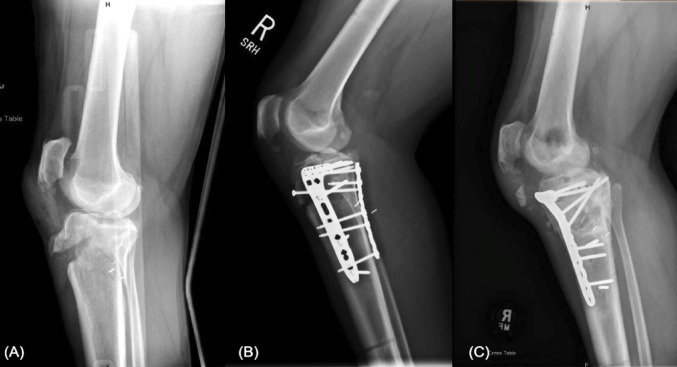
Representative case of fixation failure after operative treatment of a tibial tubercle–involving tibial plateau fracture. A Preoperative lateral radiograph. B Immediate postoperative lateral radiograph demonstrating the index fixation construct. C Follow-up lateral radiograph demonstrating construct failure and nonunion with the original hardware in situ, highlighting the significant mechanical strain on the extensor mechanism repair
